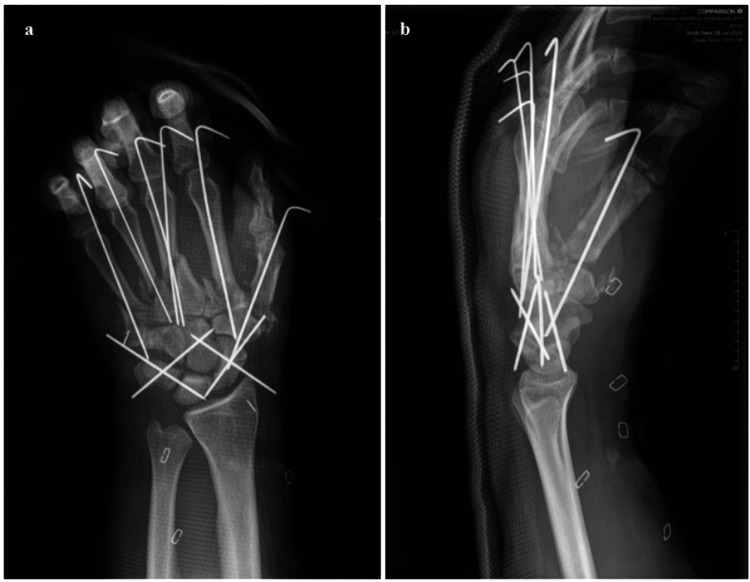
Postoperative radiographs of the left hand reveal successful stabilization of hand fractures using multiple K-wires during the primary debridement procedure. Fracture reduction and stabilization focused on restoration of the knuckle height and correcting fracture angulation, rotation, and shortening. Carpal row dissociation was addressed by employing four K-wires arranged in a diamond-shaped configuration, effectively securing the proximal and distal carpal rows while restoring Gigula’s carpal arcs. a) Left hand radiograph (posteroanterior view). b) Left hand radiograph (lateral view)
